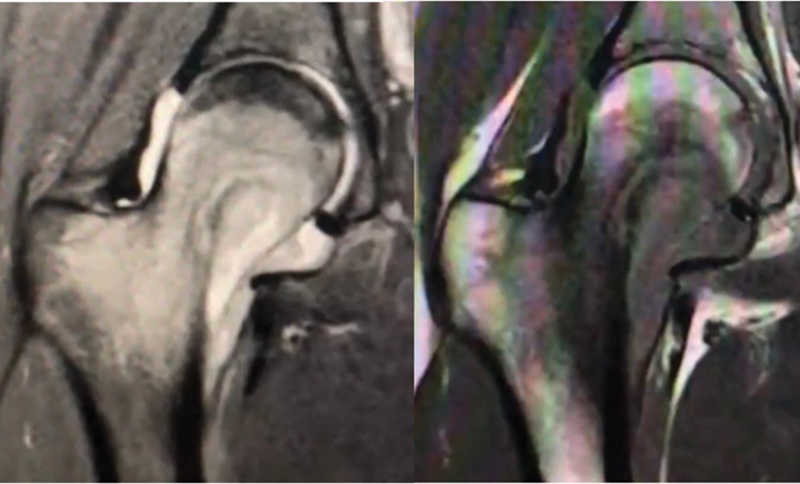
Initial Coronal MRI images, demonstrating bone marrom edema and incread intra-articular fluid.
